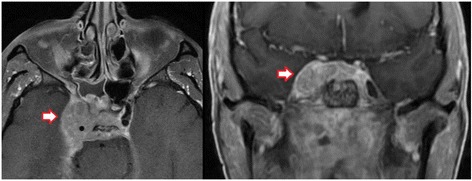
T1-weighted MRI in June of 2016 showing bulky tumor recurrence (red arrows) in axial and coronal plane
